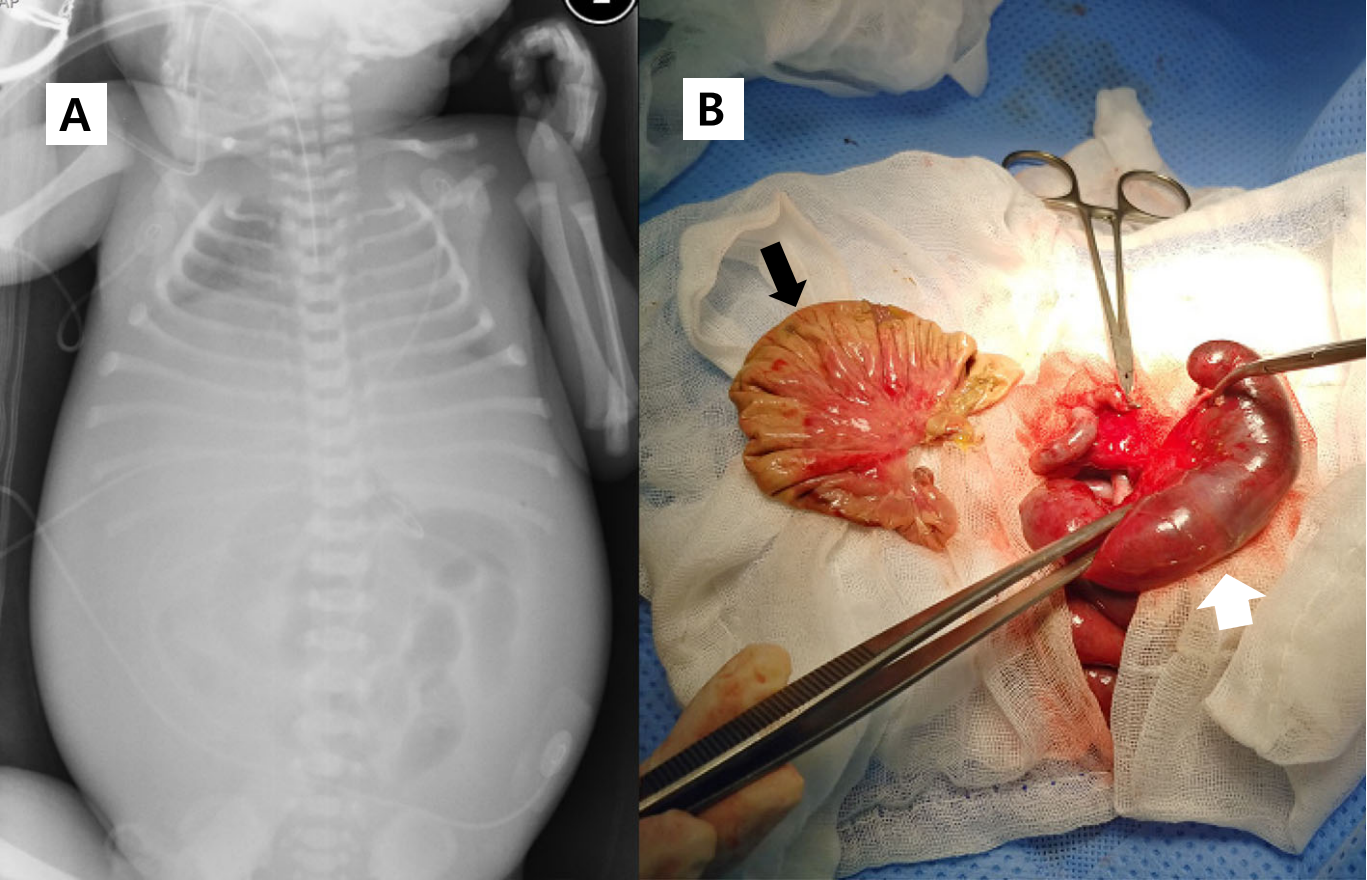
a A chest radiograph showing diffuse haziness on both lung fields and a bilaterally elevated diaphragm. b The dilated small bowel proximal to volvulus (arrow) was resected and volvus (white arrow) was seen necrotic
